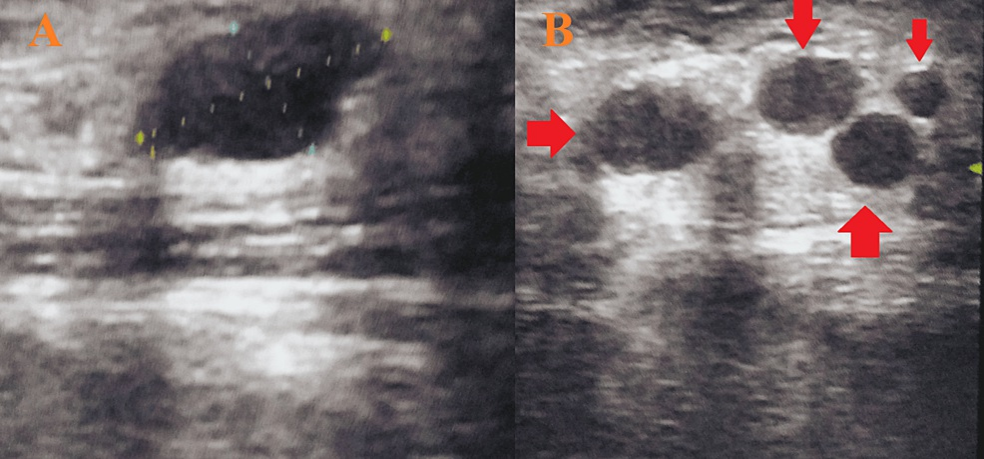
Ultrasound of the neck suggestive of multiple deep cervical lymphadenopathies on the left side A: The largest lymph node on the right side; B: Multiple deep cervical lymphadenopathies on the left side
